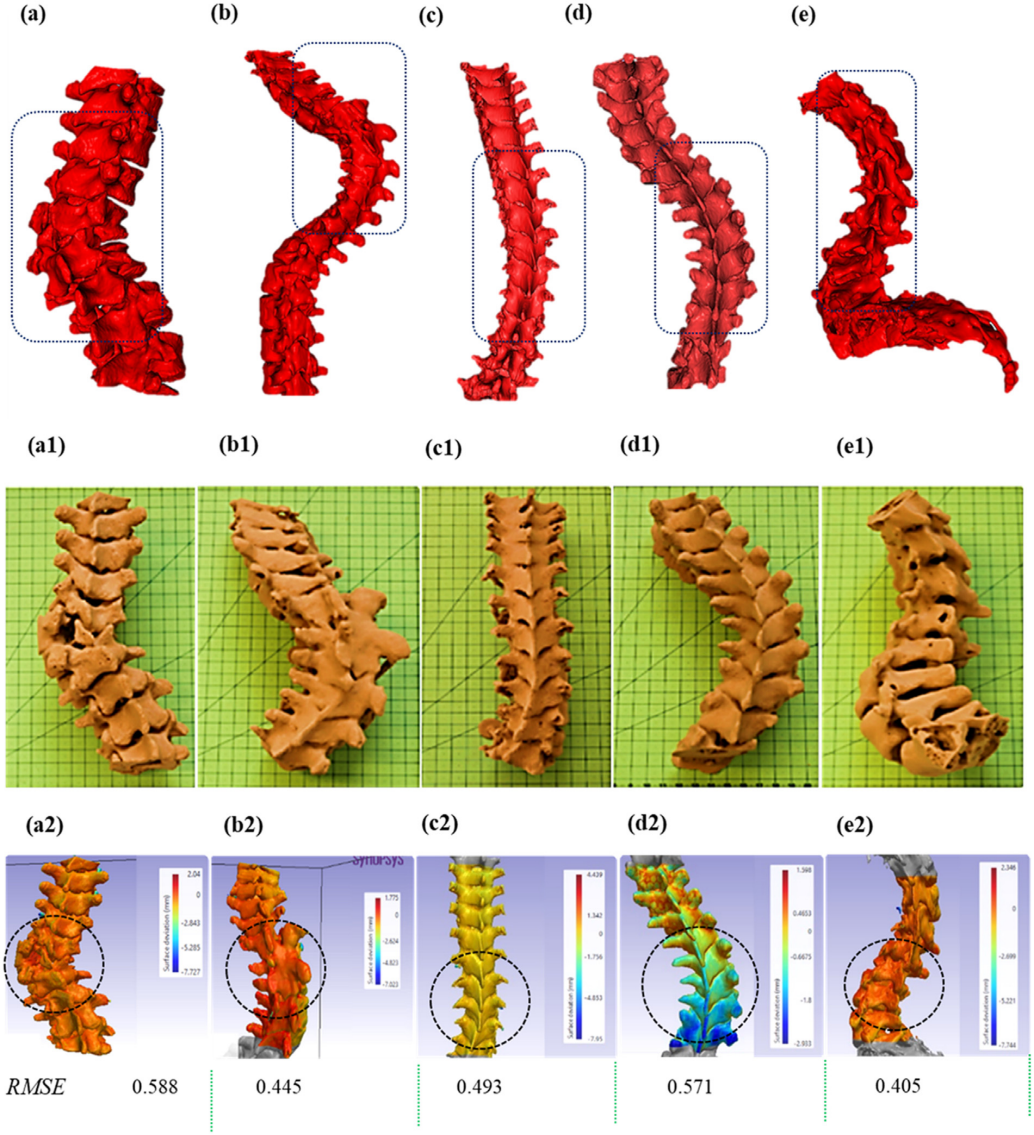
Analysis of accuracy of 3D reconstruction of the severe scoliotic spines: 3D reconstruction from corresponding CT scan dataset of patient (a) K1, (b) K4, (c) K7, (d) K10 and (e) K11; (a1 – e1)3D printed samples of the severely affected segments of the spines of the corresponding patients; (a2 – e2) surface deviation analysis of the 3D printed sections and the 3D reconstructed CAD files of the spines. *RMSE: Root Mean Square Error between the two consecutive surfaces (in mm); †Areas highlighted by dotted lines (a-e) showing the areas of severe deformations of the patients’ spines; †Areas encircled with dotted line(a2-e2) highlighting the severe areas.
